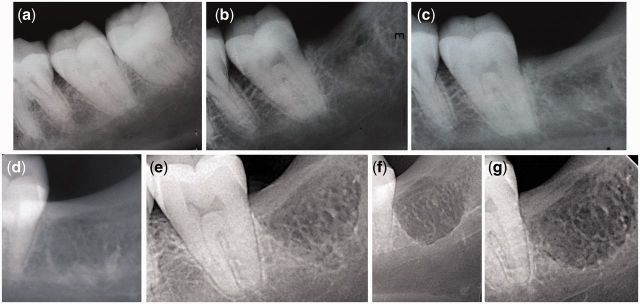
X-ray images of experimental site at different time points. (a): before extraction; (b): immediately after extraction; (c): after suturing; (d): 2 weeks after extraction; (e): 3 months after extraction; (f): 6 months after extraction; (g): 12 months after extraction.
